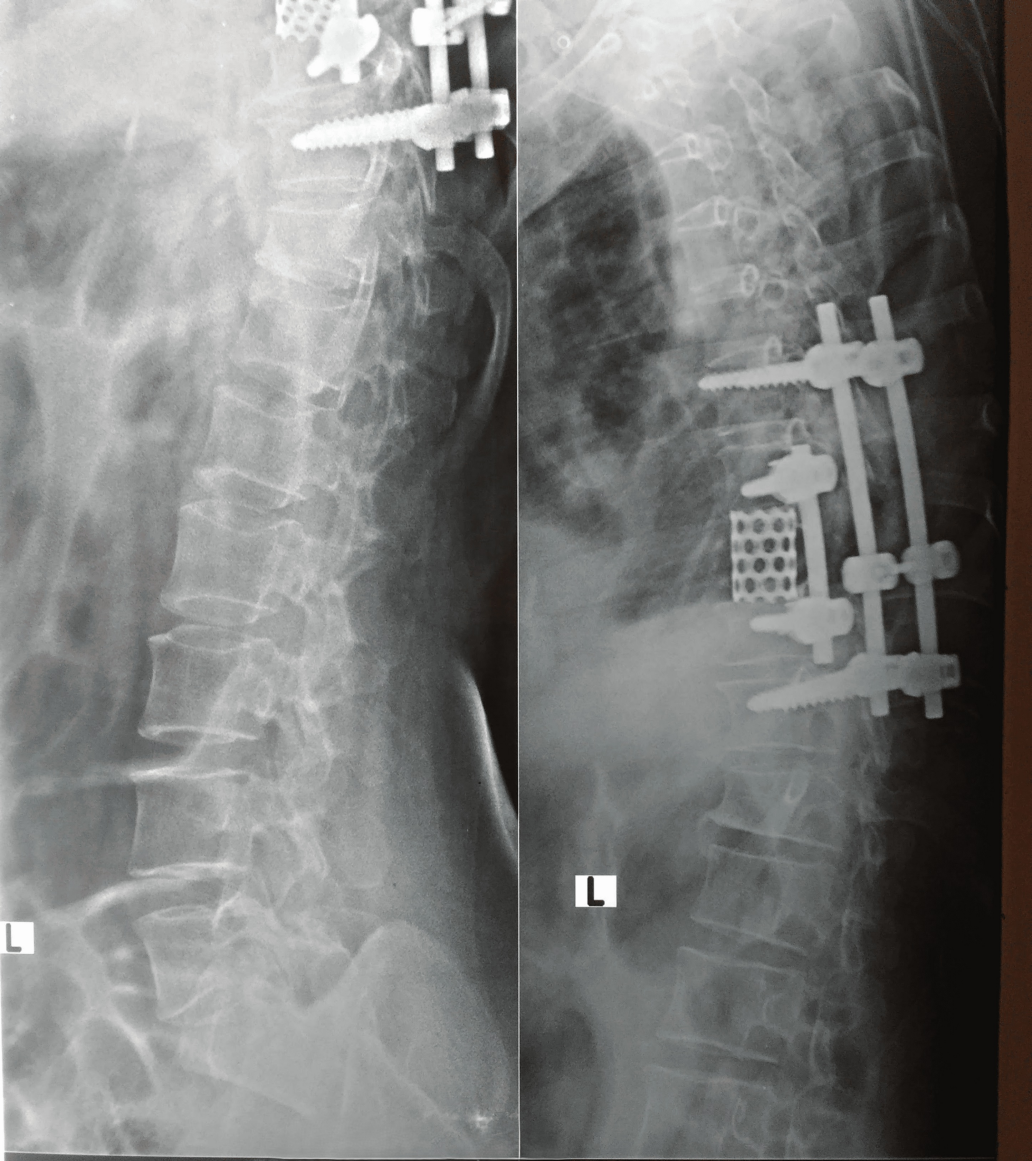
Postoperative X-ray lateral view
